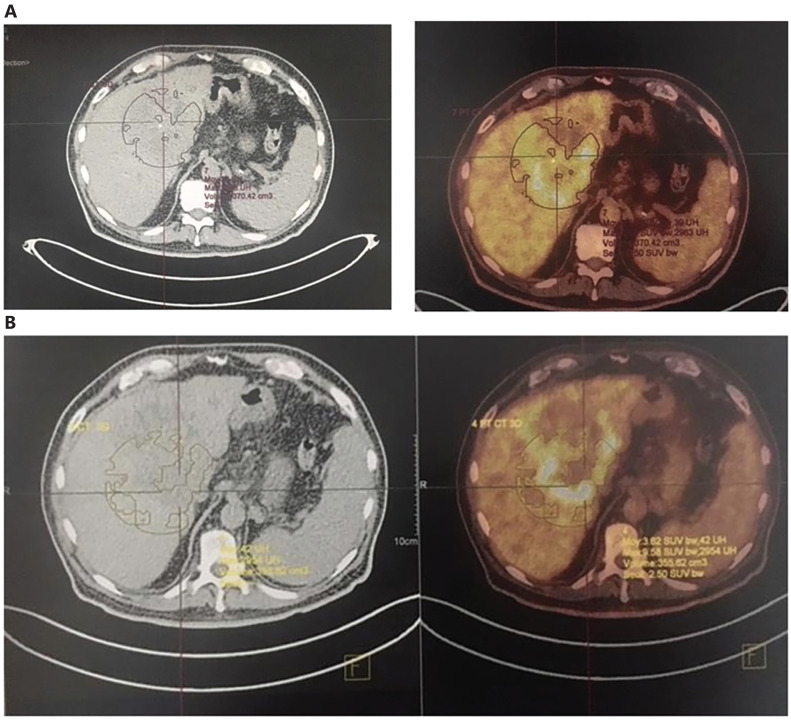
An 84-year-old man with cholangiosarcoma treated with only autologous serum vaccine (APAVAC^®^), A: CT-scan and FDG pet-scan before treatment and B: evaluation after 4 doses of sub cutaneous injection of APAVAC^®^ once per week.
